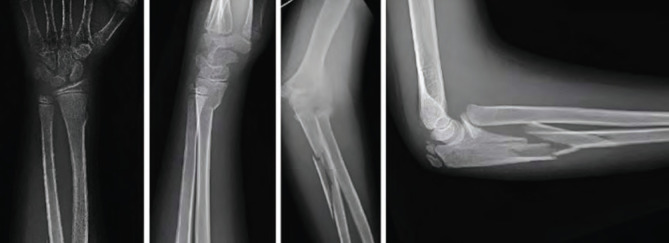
Pre-operative anteroposterior and lateral radiographs showing a Monteggia equivalent fracture characterised by a comminuted fracture of the proximal ulnar diaphysis and a radial neck fracture accompanied by an ipsilateral distal radius fracture.
